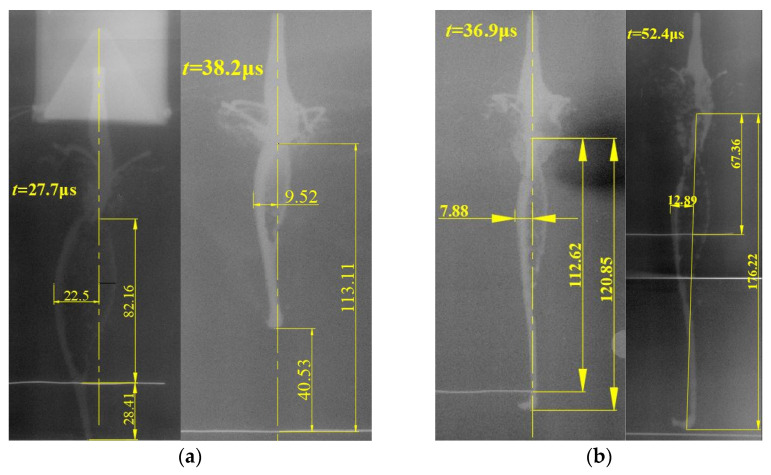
X-ray image of the SCJ from the shaped charge with a trapezoid cross-section. (a) SCJ produced by the shaped charge with an acute angle of 60°, (b) SCJ produced by the shaped charge with an acute angle of 75°.
